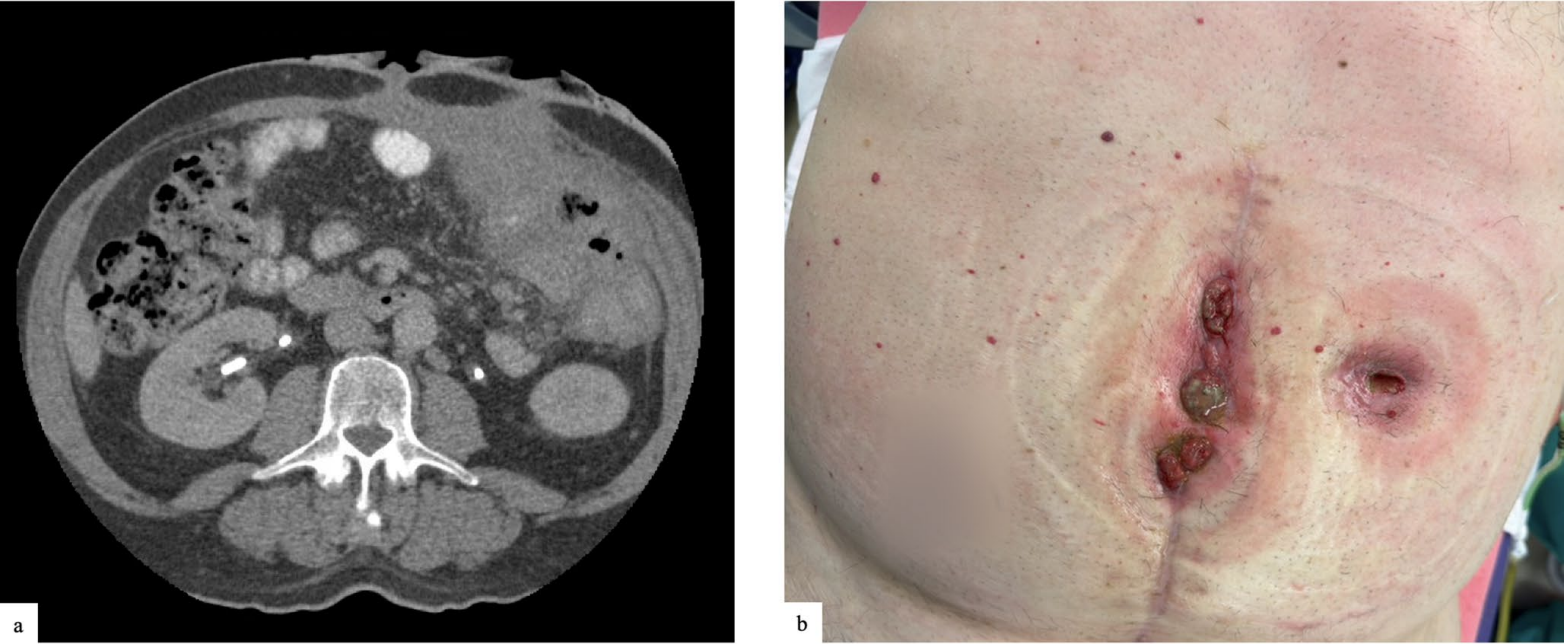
shows a 60-year-old man with a history of Crohn’s disease and multiple enterocutaneous fistulae presenting for fistula takedown, incisional hernia repair and ostomy reversal, (a) shows the preoperative axial CT scan with evidence of multiple fistula and significant hernia (b) preoperative clinical photographs of wounds
